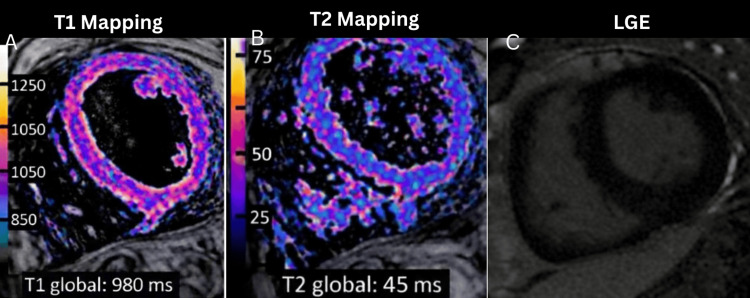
Cardiac MRI Tissue Characterization (T1, T2, and LGE Mapping) A: The native T1 parametric map demonstrates a global T1 value of 980 ms. At a field strength of 1.5T, the typical reference range for healthy myocardium is approximately 950–1050 ms. The myocardium exhibits a homogeneous signal without evidence of significant T1 prolongation. Under the Lake Louise Criteria, a normal native T1 value suggests the absence of diffuse interstitial expansion, which would typically be seen in the presence of myocardial edema or fibrosis. The pixel-wise map shows no focal areas of increased T1, consistent with normal myocardial tissue composition. B: Quantitative T2 mapping reveals a global T2 value of 45 ms. The established reference range for normal myocardium at 1.5T is generally 40–50 ms. There is no evidence of myocardial edema, as the T2 values remain well below the pathological threshold (typically >52-55 ms at 1.5T). The color-coded map confirms a uniform distribution of T2 relaxation times across the short-axis slice, with no focal "hot spots" in the subepicardial or mid-wall layers. This represents a negative T2-based criterion for active inflammatory edema. C: Post-contrast T1-weighted inversion recovery imaging shows no evidence of late gadolinium enhancement. There are no visible areas of focal hyperenhancement in the subepicardial or intramural distributions, which are the hallmark signatures of myocardial necrosis or replacement fibrosis in myocarditis. This finding satisfies the negative T1-based criterion for focal myocardial injury.
